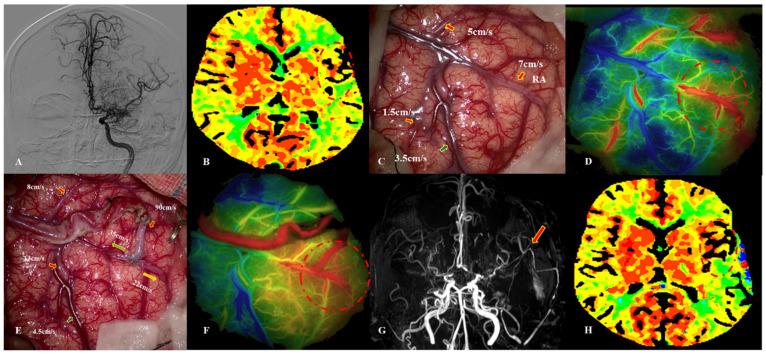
This 39-year-old female patient was diagnosed with MMD due to paresthesia. Intraoperative image of MDU hemodynamic analysis in PSCAs. (A) DSA image before surgery. (B) CTP showed that TTP was delayed (dotted red circle). (C) Hemodynamics of PSCAs before anastomosis (artery: red arrow, vein: blue arrow). RA was chosen from PSCAs with MVV of 7 cm/s. (D) Blood distribution by FLOW 800 image before bypass (dotted red circle). (E) MDU hemodynamics of PSCAs after bypass, with a 5-fold increase for RA.ES (green arrow) and a 3-fold increase for RA.LS (orange arrow). (F) Postoperative hemodynamics evaluated by FLOW 800 image, indicating that regional perfusion improved (dotted red circle). (G) MRA demonstrated patency of donor artery (red arrow). (H) Postoperative CTP images showed that regional TTP decreased. This patient presented with severe headache and verbal dysfunction at 3 days after bypass. MDU = microvascular doppler ultrasonography, PSCAs = para-sylvian cortical arteries, CHS = cerebral hyperperfusion syndrome, RA.ES = recipient artery entering sylvian, RA.LS = recipient artery leaving sylvian.
